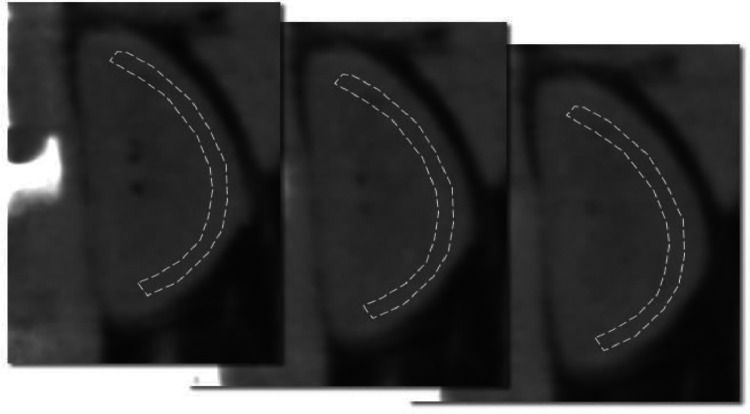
Three renal cortex ROIs are shown. The average of the three renal cortex ROIs was used as the raw CT HU for that kidney. The ROIs excluded medulla. They did not include cystic areas. There was no case showing hydronephrosis.
